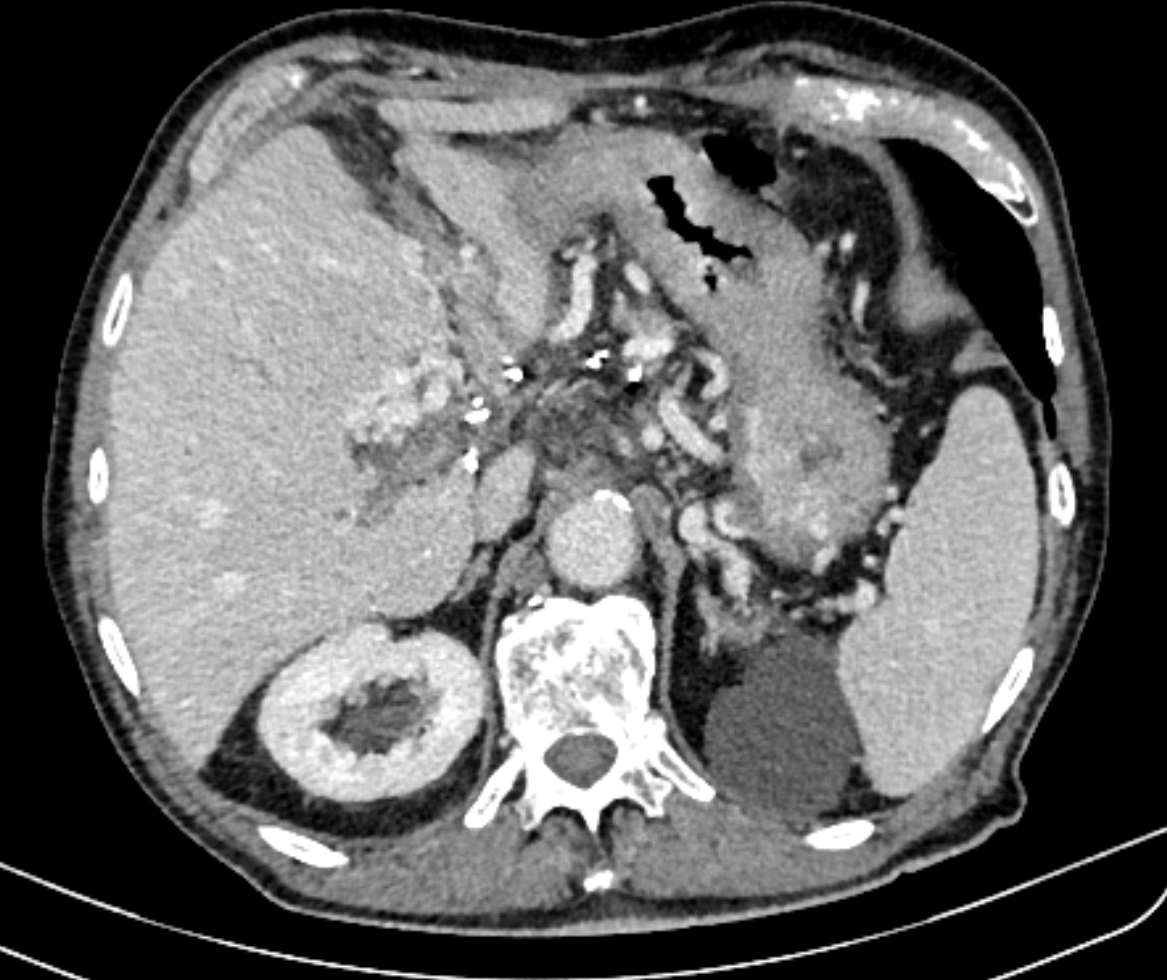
CT scan showing thrombosis of portal, splenic and superior mesenteric vein with a probable tumor thrombus.
